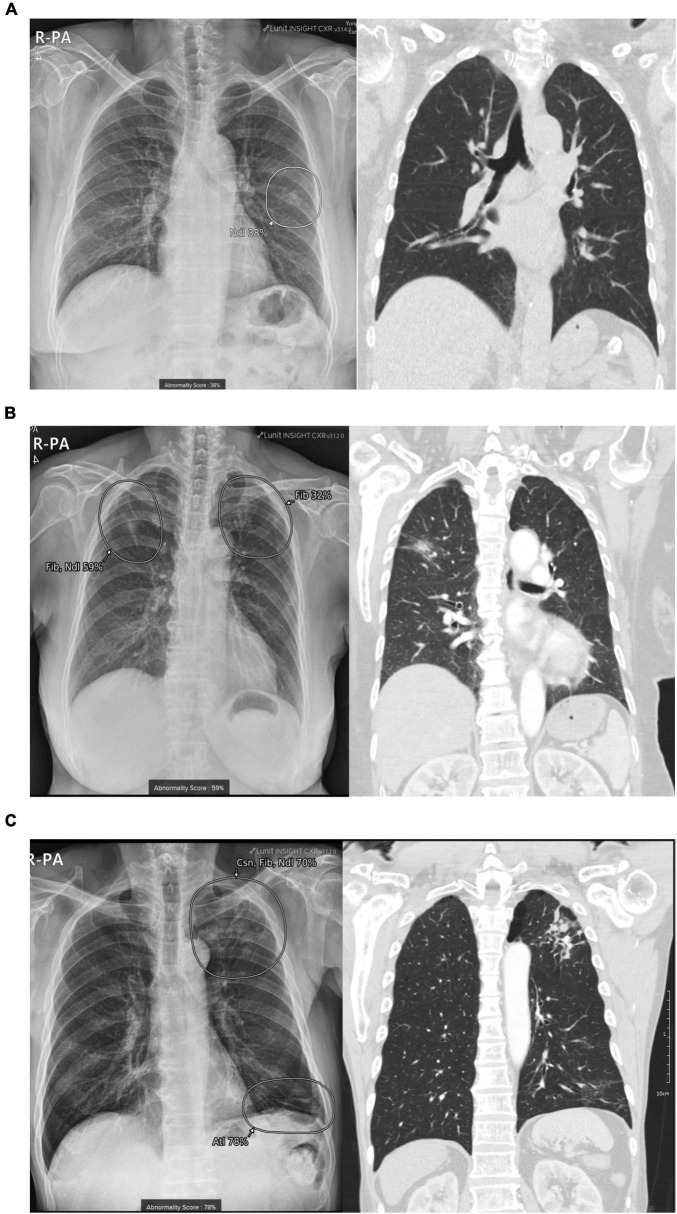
Examples of patient cases. (A) False-positive nodule detected on CXR by AI: A 75-year-old man underwent a CXR examination at the cardiology outpatient clinic, which suggested a nodule in the left middle lung field (AI nodule score: 38%). Subsequent chest CT revealed no significant abnormalities, and the nodule was identified as a rib or a vascular shadow. (B) True-positive nodule detected on CXR by AI, lung cancer: A 71-year-old woman underwent a preoperative examination for spinal surgery, which suggested fibrosis combined with a nodule in the right upper lung field. A subsequent chest CT scan revealed an approximately 3 cm part-solid nodule. The final pathological diagnosis after surgery confirmed stage IA adenocarcinoma (Nodule score: 59%; Fibrosis score: 26%). (C) True-positive nodule detected on CXR by AI and acute pulmonary infection: A 75-year-old female patient underwent CXR at the gastroenterology outpatient clinic, where AI suggested combined findings of consolidation, fibrosis, and a nodule in the left upper lung field. Subsequent chest CT revealed multiple centrilobular nodules, and a sputum test was performed to diagnose active pulmonary TB (AI Nodule score, 54%; consolidation score, 24%; fibrosis score, 70%). AI, artificial intelligence; CXR, chest radiograph; Ndl, nodule; Csn, consolidation; Fib, fibrosis.
